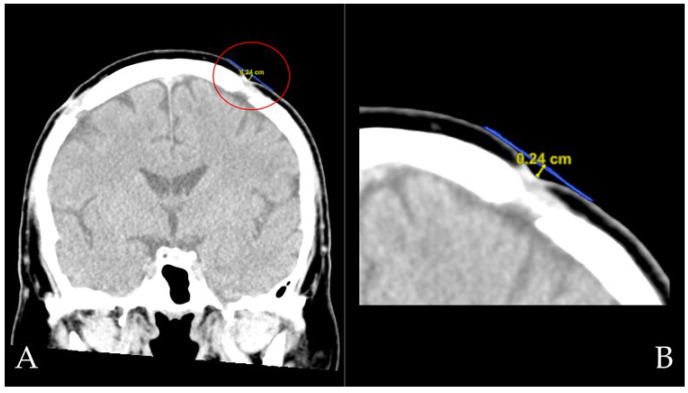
(A) A coronal CT image showing how radiological scalp depressions were measured, with an encircled scalp depression. (B) A magnified image of the scalp depression, to highlight how the distance was measured from the bottom of the depression to the hypothetical scalp line.
